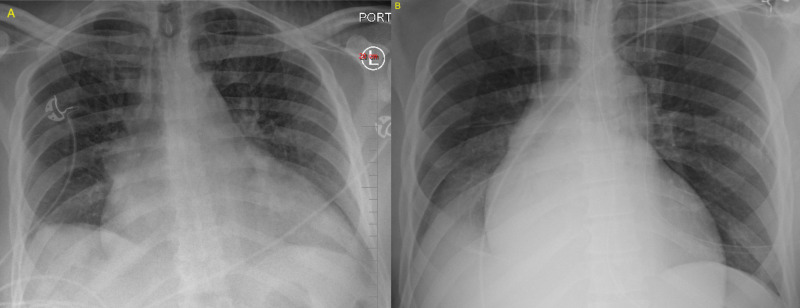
Chest Radiography on Admission and Prior to Discharge in a Patient with Asymptomatic COVID-19 Chest radiography on admission showed cardiomegaly, the cephalization of pulmonary vasculature from congestive heart failure, and no infiltrate (A). Chest radiography prior to discharge showed cardiomegaly, improved pulmonary vascular congestion, and no infiltrate (B).
